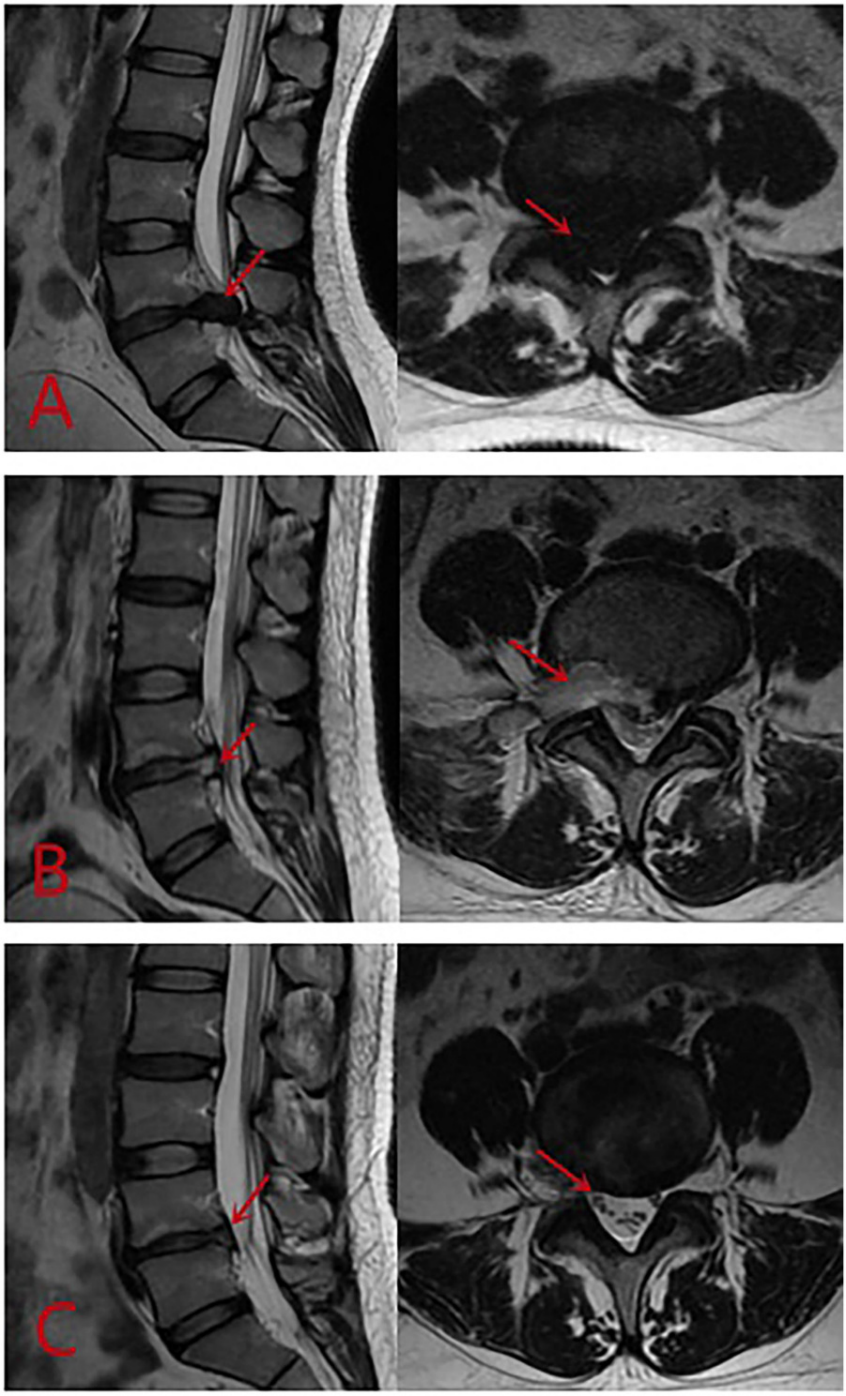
Preoperative MRI T2-weighted image showing a large central L5-S1 disc herniation causing severe compression of the cauda equina (A), which was completely removed on the first postoperative day (B), and the restoration of spinal canal volume to normal at 3 months after non-pressurized PETD surgery (C).
